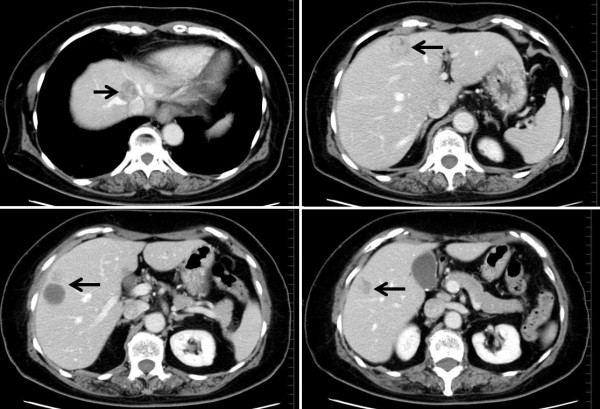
Abdominal contrast-enhanced CT findings upon admission. Arrows indicate the positions of four heterogeneous liver tumors.
